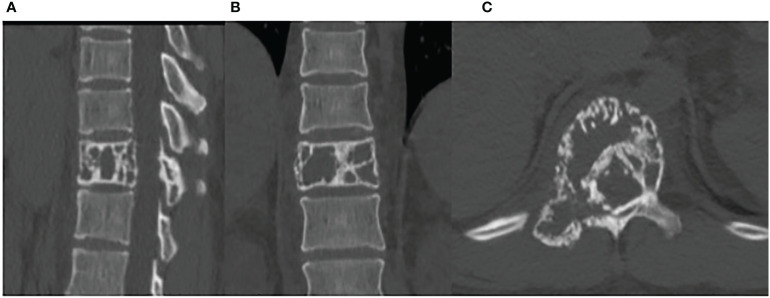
The CT demonstrates bone lesions located in the T11 vertebral body. (A) sagittal image. (B) coronal image. (C) axial image.
